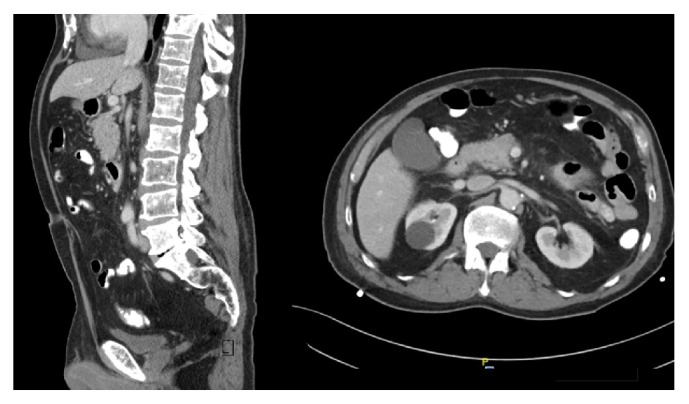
CT chest and abdomen/pelvis with contrast. Diffuse heterogeneous marrow signal of the thoracic spine and lumbar spine (left). Right kidney has several cysts with the largest measuring 2.8 x 2.9 cm (right). Findings were suggestive of diffuse osteopenia versus bony metastasis.
